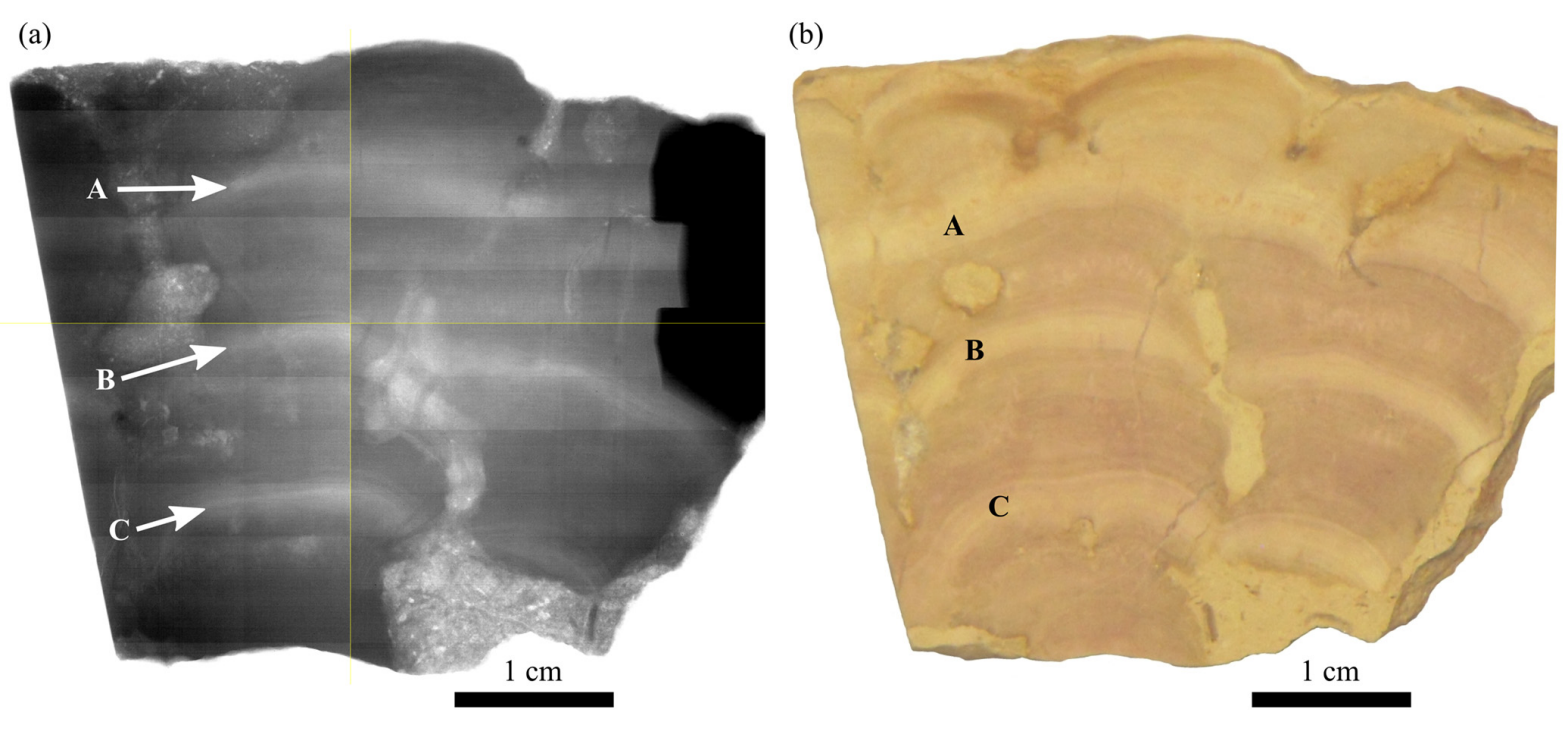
2D X-ray radiograph of UOM-232 (a) showing differences in calcite density in the different visible bands (b). Letters indicate equivalent bands on both images. The white bands are lower density than the pink bands.
